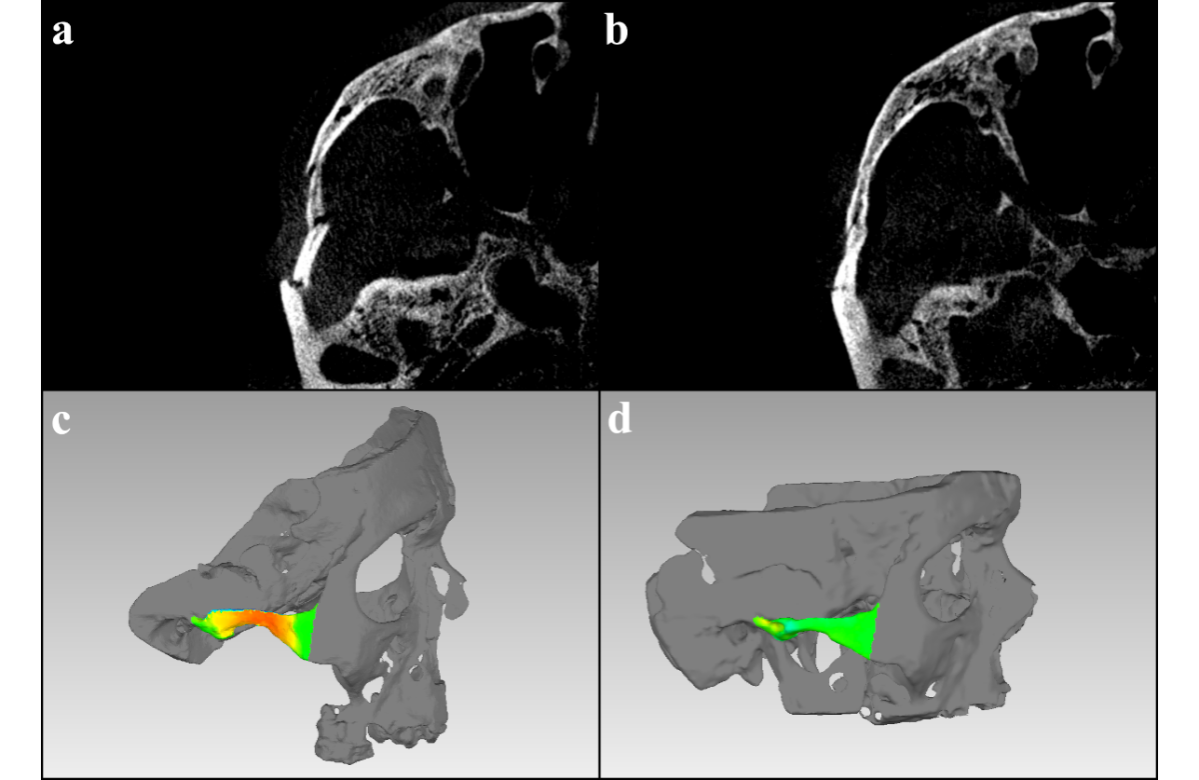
Evaluation: (a) A fractured zygomatic arch visualized before reduction and (b) after reduction in axial cone beam computed tomography slices. (c) The deviation of a fractured zygomatic arch is displayed in color in Geomagic Studio 2013 (3D Systems Inc). Red is for severe deviation (≥1 mm) and green for minor deviation (<1 mm). (d) The same case in Geomagic Studio 2013 after reduction.
